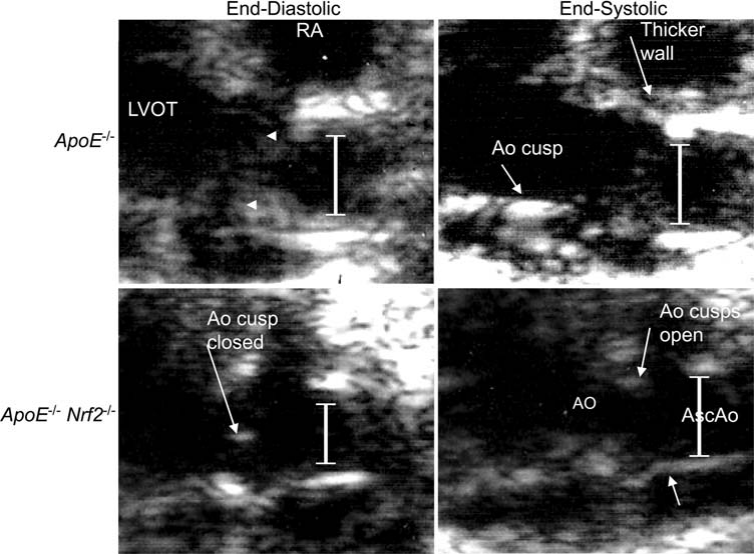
Representative 2 D mode echocardiogram images from *ApoE*
